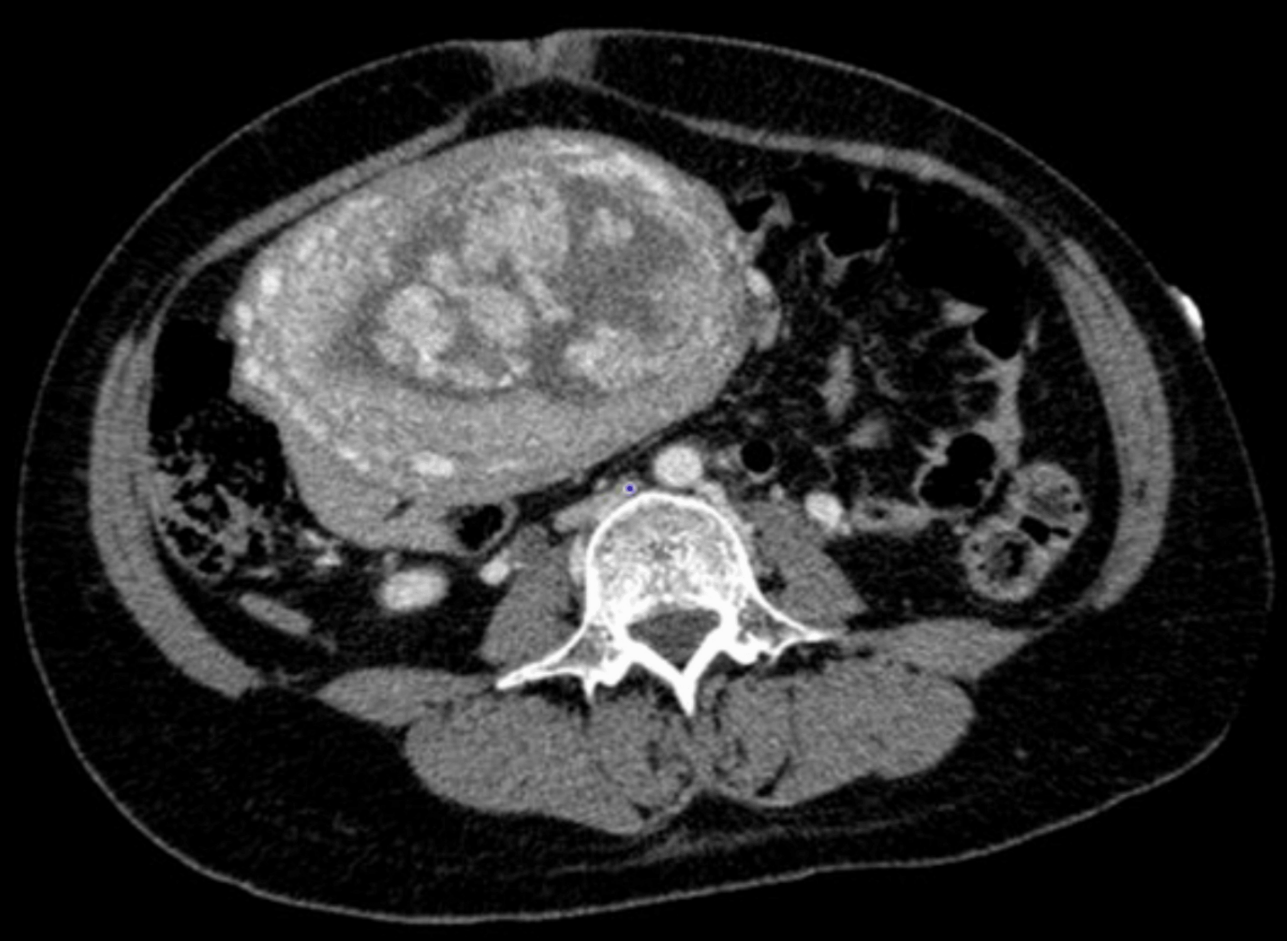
Computed tomography scan (axial section) The placenta is adherent to the uterine fundus. There is no leakage of the contrast medium into the uterine cavity.
